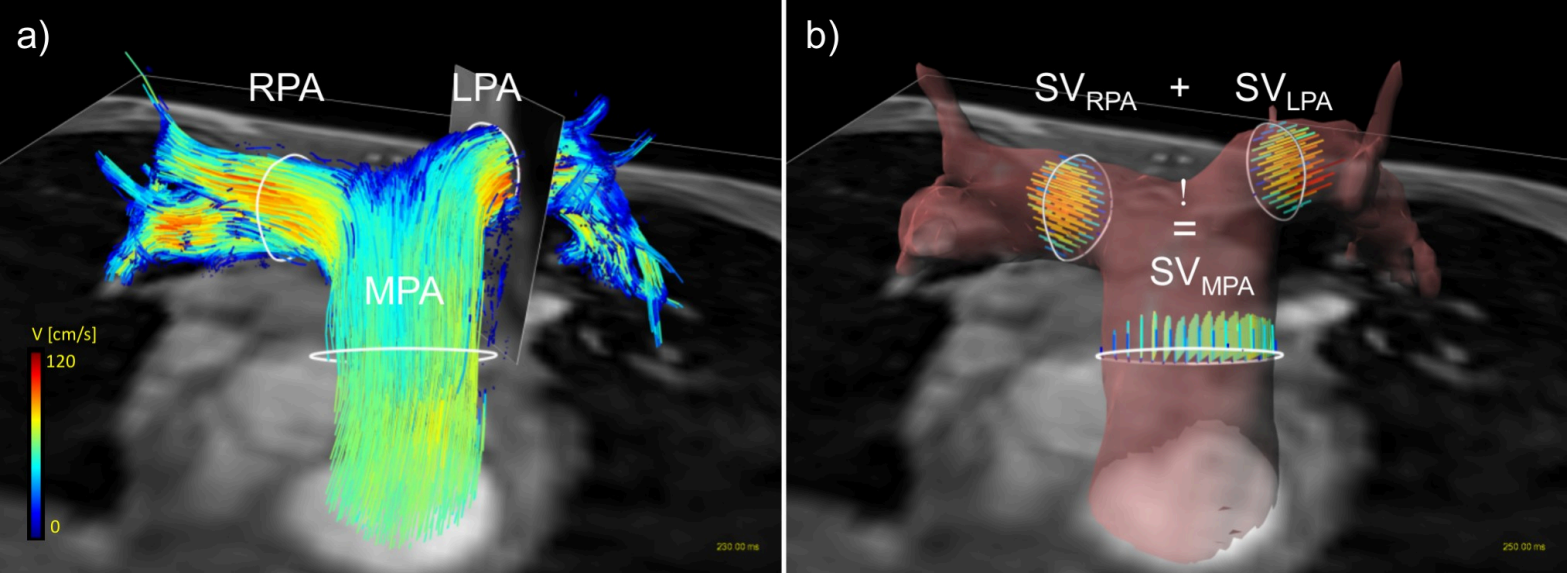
Data analysis of a 4D Flow MRI dataset. Fig 1 a) shows contour-placement (white) in the main, right and left pulmonary artery (MPA, RPA, LPA, respectively) in a healthy volunteer. The oblique cutplane transecting the LPA matches the 2D Flow geometric orientation to guarantee the exact comparability of data. Visualization in (a) was achieved by using particle-traces, projected in both flow directions, with contours as seed points. Color-coding was performed with respect to the acquired velocities. (b) the principle of the Conservation of mass-analysis in the same volunteer. Stroke volume in the MPA should equal the sum of stroke volume in the RPA and LPA to proof data consistency. The vessel wall was reconstructed based on complex difference and surface shading of the 4D Flow MRI data.
